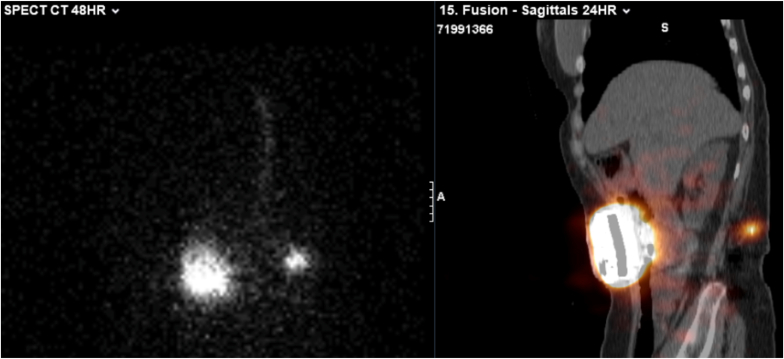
SPECT/CT Localization of Intrathecal Catheter Leak Planar gamma camera images and SPECT/CT of the lower abdomen and pelvis were obtained at 24 and 48 h following In-111 DTPA injection into the intrathecal pump reservoir. SPECT/CT demonstrates focal radiotracer activity at the L3 level, corresponding to the catheter transition from subcutaneous tissue through the musculature before entering the spinal canal, consistent with a catheter leak. Mild activity is also visualized within the intrathecal portion of the catheter.
